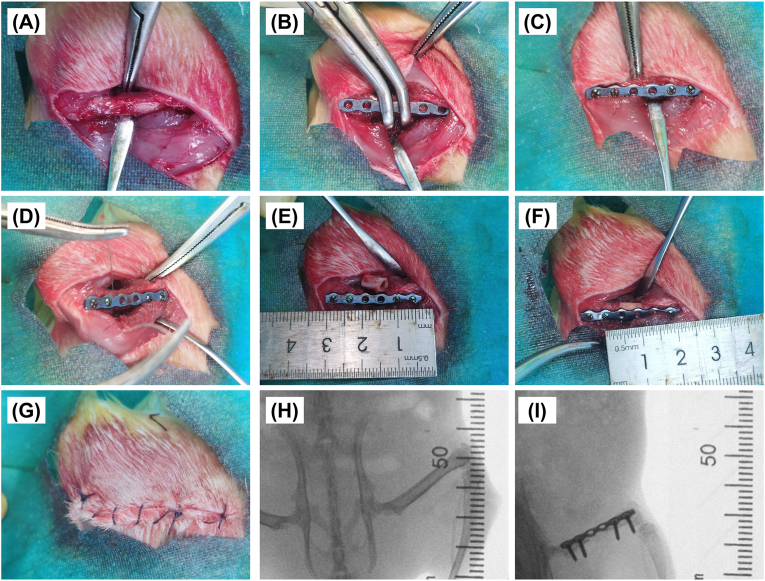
Surgical procedure. (A) Exposure of the femur. (B) Initial fixation. (C) Screw fixation. (D–E) Creation of the defect with a wire saw. (F) Implantation of a matrix scaffold. (G) Completion of sutures. (H) Preoperative x-ray. (I) Postoperative x-ray.
